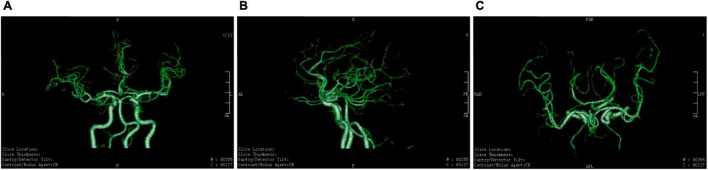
Cerebral vessels in 2011. (A–C) Head computed tomography angiography findings in 2011. His cerebral vessels ran normally, and there was no stenosis of the lumen.
